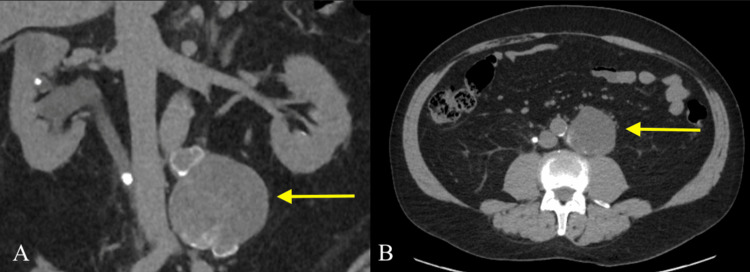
Coronal and axial non-contrast CT images of the abdomen and pelvis. Coronal image (A) demonstrates mild hydronephrosis of the right kidney secondary to an obstructing 9 mm calculus in the mid-right ureter. Incidentally, detected a 7.7 cm partially calcified mass in the left periaortic retroperitoneum is seen on axial image (B) as well (arrows).
